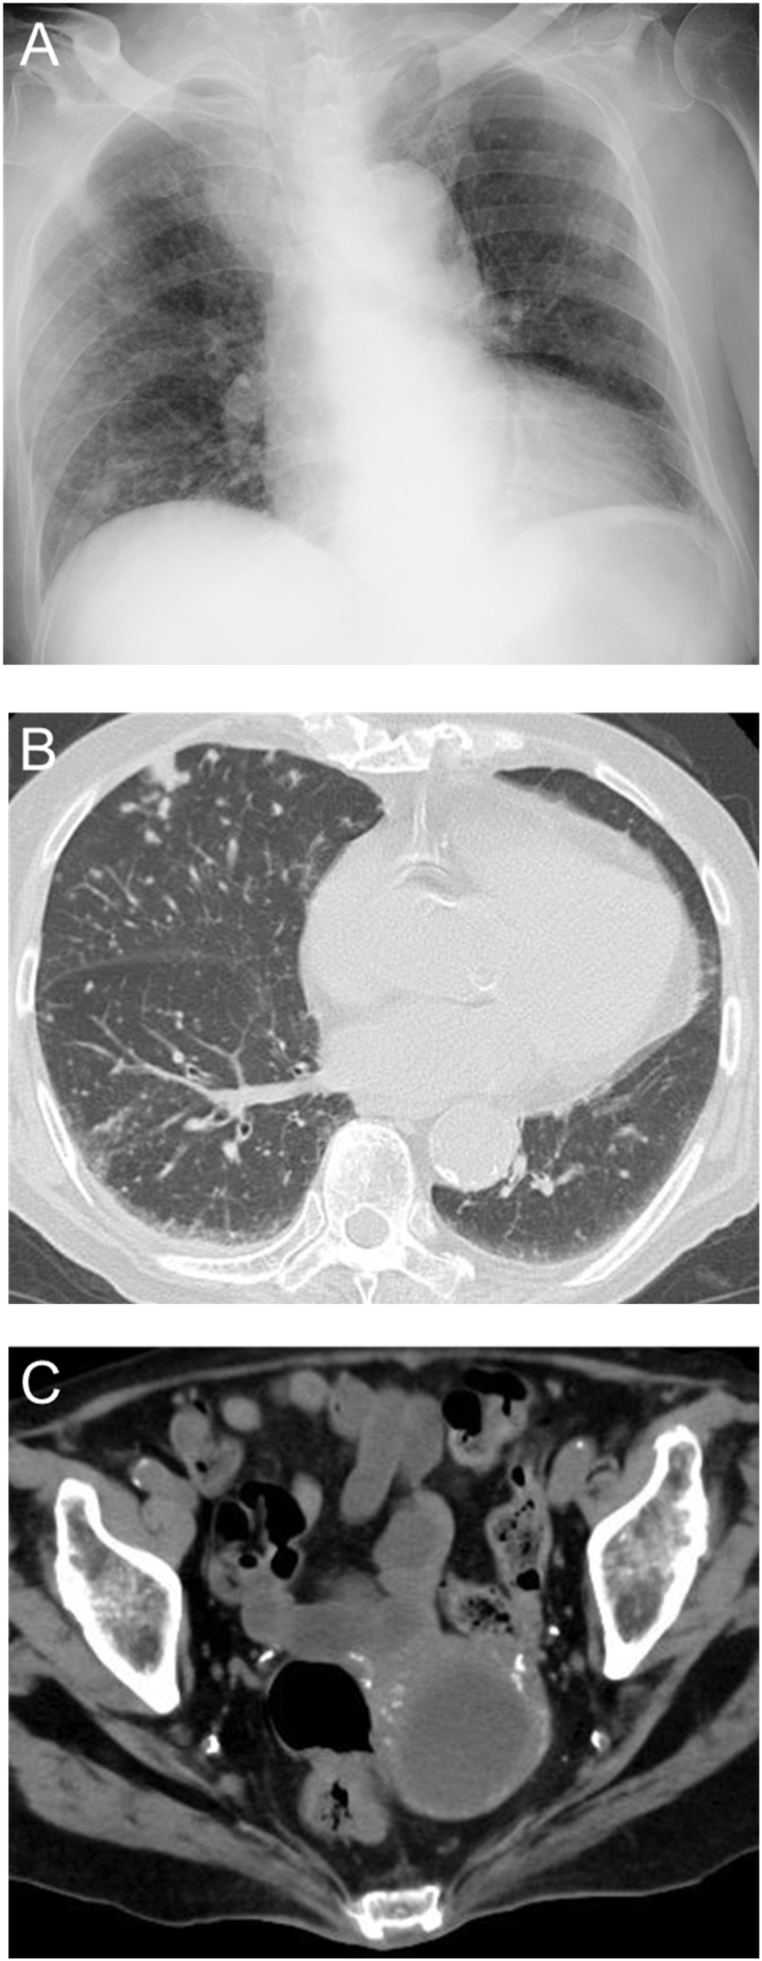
CT findings on admission to our hospital. A. Chest CT demonstrated diffuse, bilateral, granular opacities. B. Abdominal and pelvic CT demonstrated an enlargement of the uterine pyometra.
